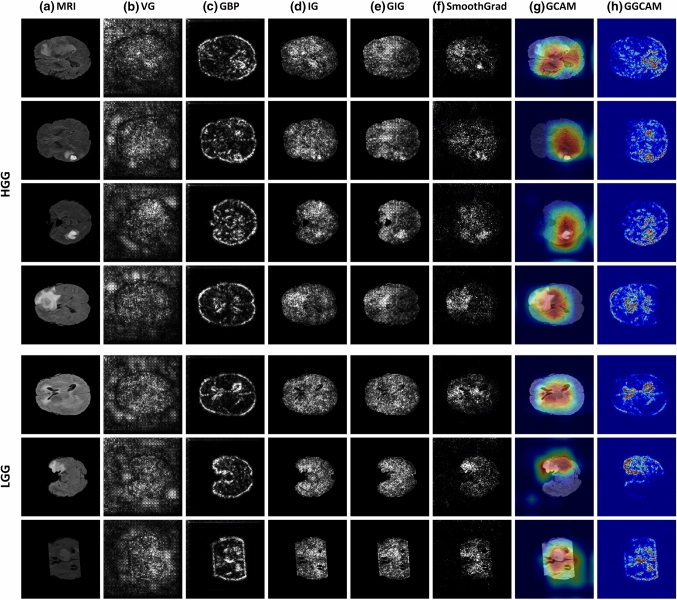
Comparing different XAI visualization methods for brain glioma classification. Sensitivity maps are presented for HGG cases in the first four rows, while for LGG cases in the last three rows. Left to right: original MRI image, Vanilla gradient, guided backpropagation, integrated gradients, guided integrated gradients, SmoothGrad, Grad-CAM, and guided Grad-CAM visualizations. Note that in (b, c, d, e, f), all contributing features are highlighted in white, while in (g, h), red regions correspond to a high score for the predicted class
